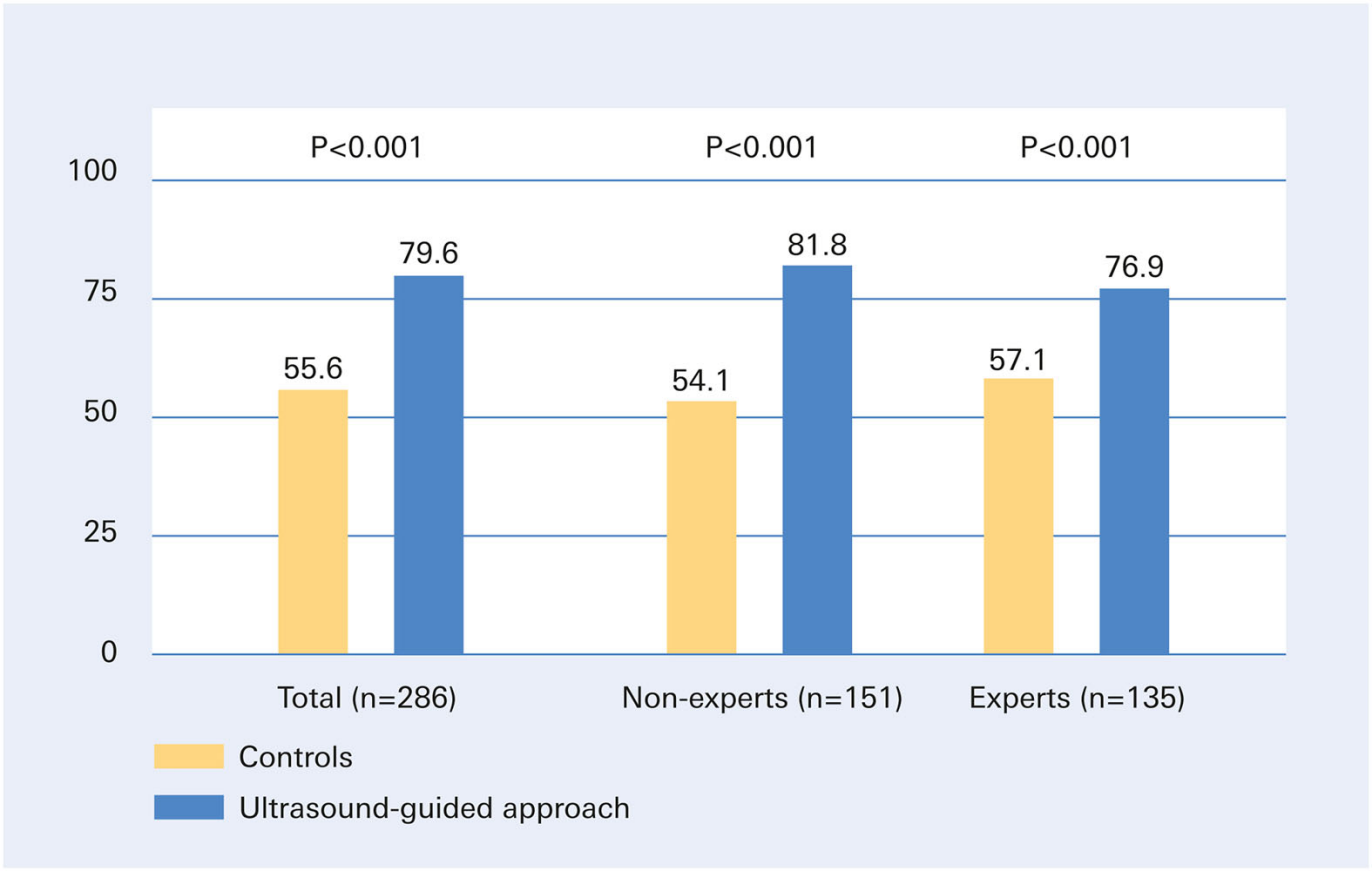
Successful puncture of the common femoral artery on the first attempt. Primary successful puncture of the common femoral artery (primary endpoint of the PARFEM trial) was achieved significantly more often in the 142 patients punctured with ultrasound guidance than in the 144 controls (left columns). The benefit of ultrasound‐guided puncture was independent of the examiners’ level of femoral puncture experience and was evident in both “non‐experts” (middle columns) and “experts” (right columns). Values expressed as percentages. [Color figure can be viewed at wileyonlinelibrary.com]
